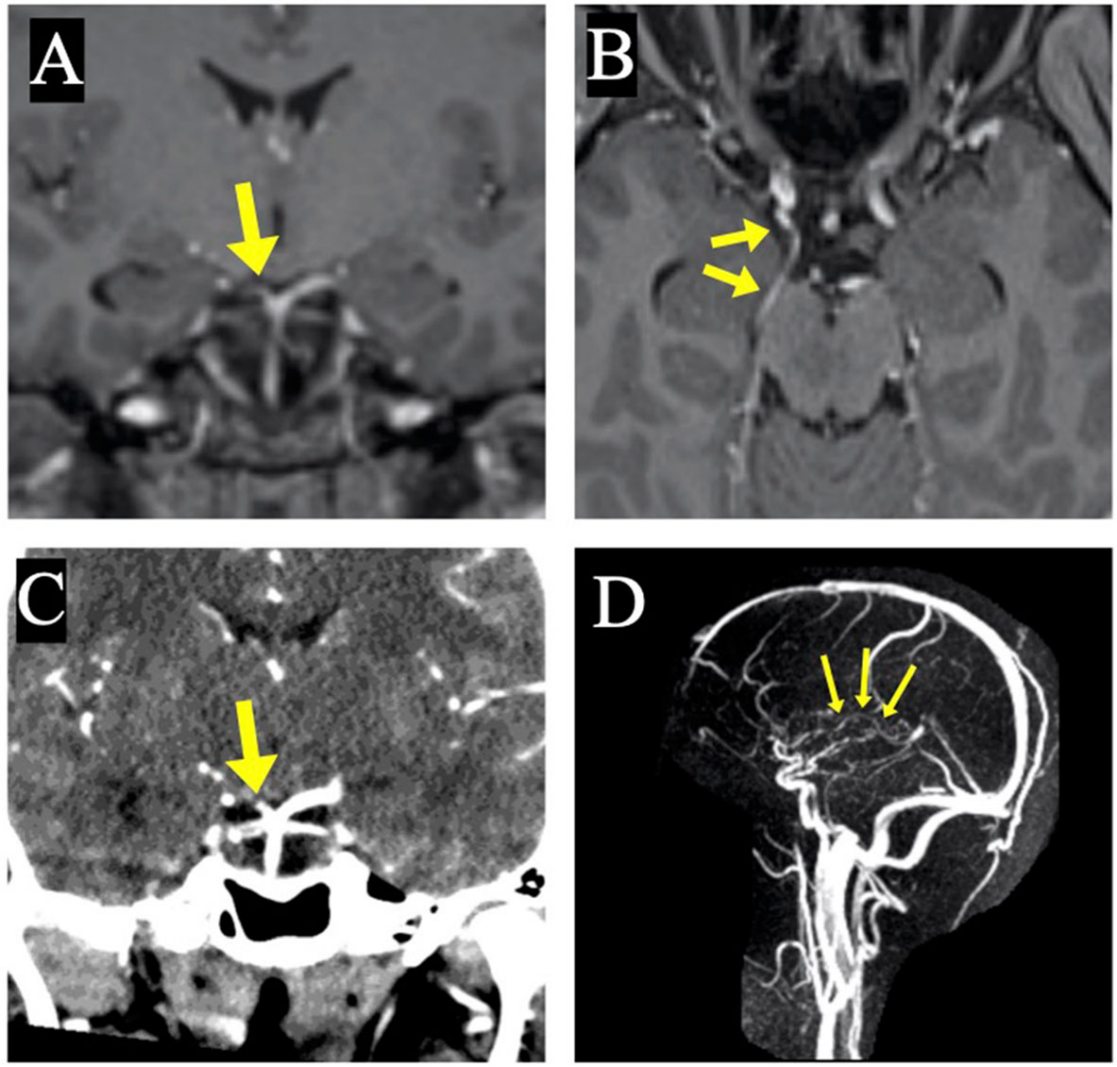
Angiographic findings suggestive of occlusion of right artery of Percheron. Contrast-enhanced magnetic resonance imaging (a) and CT angiogram (c) demonstrated normal opacification and diameter of the vertebrobasilar arterial trunk, excluding top of the basilar artery syndrome. There was slight asymmetry of the posterior cerebral arteries (PCAs) with relative narrowing of the right P1 segment compared to the left (yellow arrows in a and c), raising the possibility of incomplete P1 occlusion or stenosis and occlusion at the level of the perforating branches of the right PCA, namely artery of Percheron. However, the filling of the more distal part of the right PCA appeared normal, likely due to the contribution from a robust posterior communicating artery (yellow arrows in b). Magnetic resonance venogram (d) demonstrated normal opacification and filling of the internal cerebral veins (yellow arrows) and intracranial venous sinuses, excluding deep cerebral venous infarct
